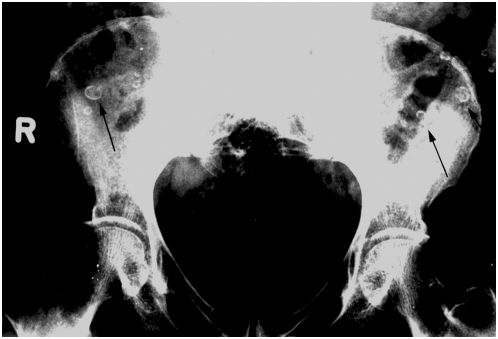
Radiograph of the pelvis from female patient with chronic lower abdominal pain. Calcified *Armillifer* nymphs (arrows) are shown in front of the iliac bones of a Nigerian patient. (Radiograph by courtesy of Klaus-J. Volkmer, Germany)
